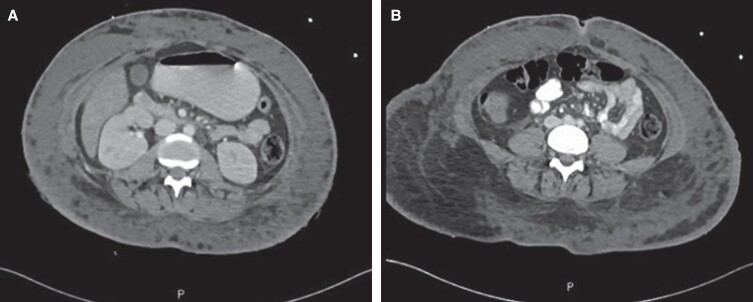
The axial computed tomography scans of a 29-year-old female showing (A) significant fat stranding and (B) edematous changes in the abdominal wall.
